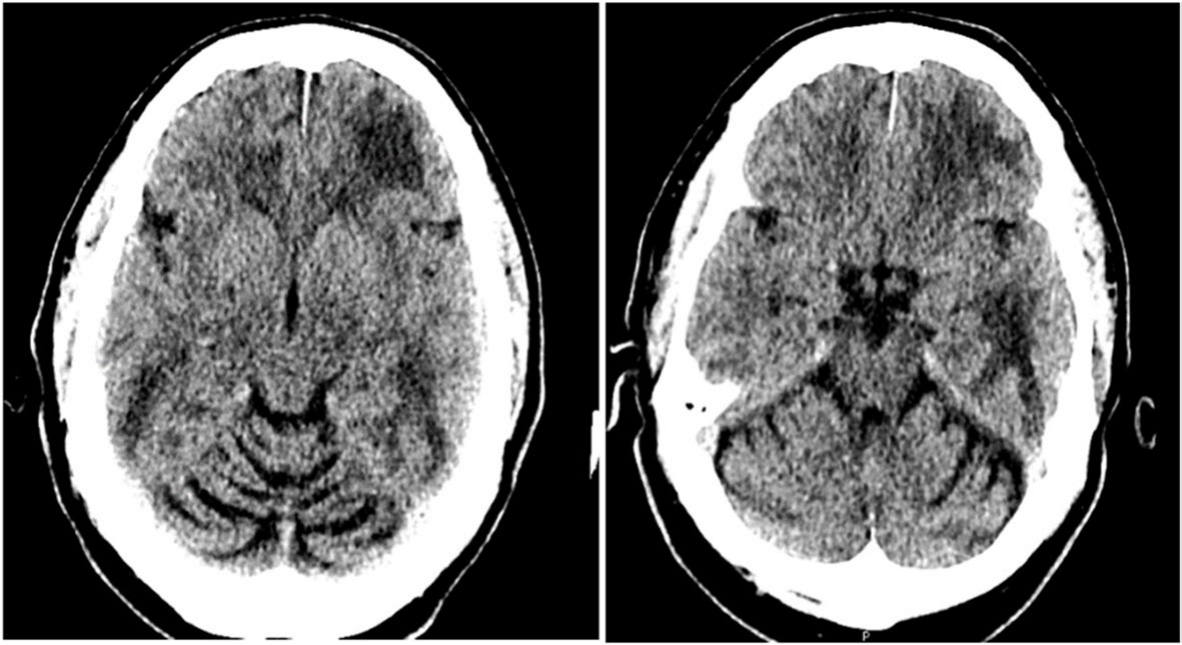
CT without contrast, initial scan on presentation. This head CT revealed confluent areas of white matter hypoattenuation bilaterally, slightly worse on the left, involving the subcortical white matter. No significant mass effect midline shift, or hemorrhage. The differential was thought to include leukoencephalopathy, vasculitis, or infiltrating neoplasm such as lymphoma among other etiologies
